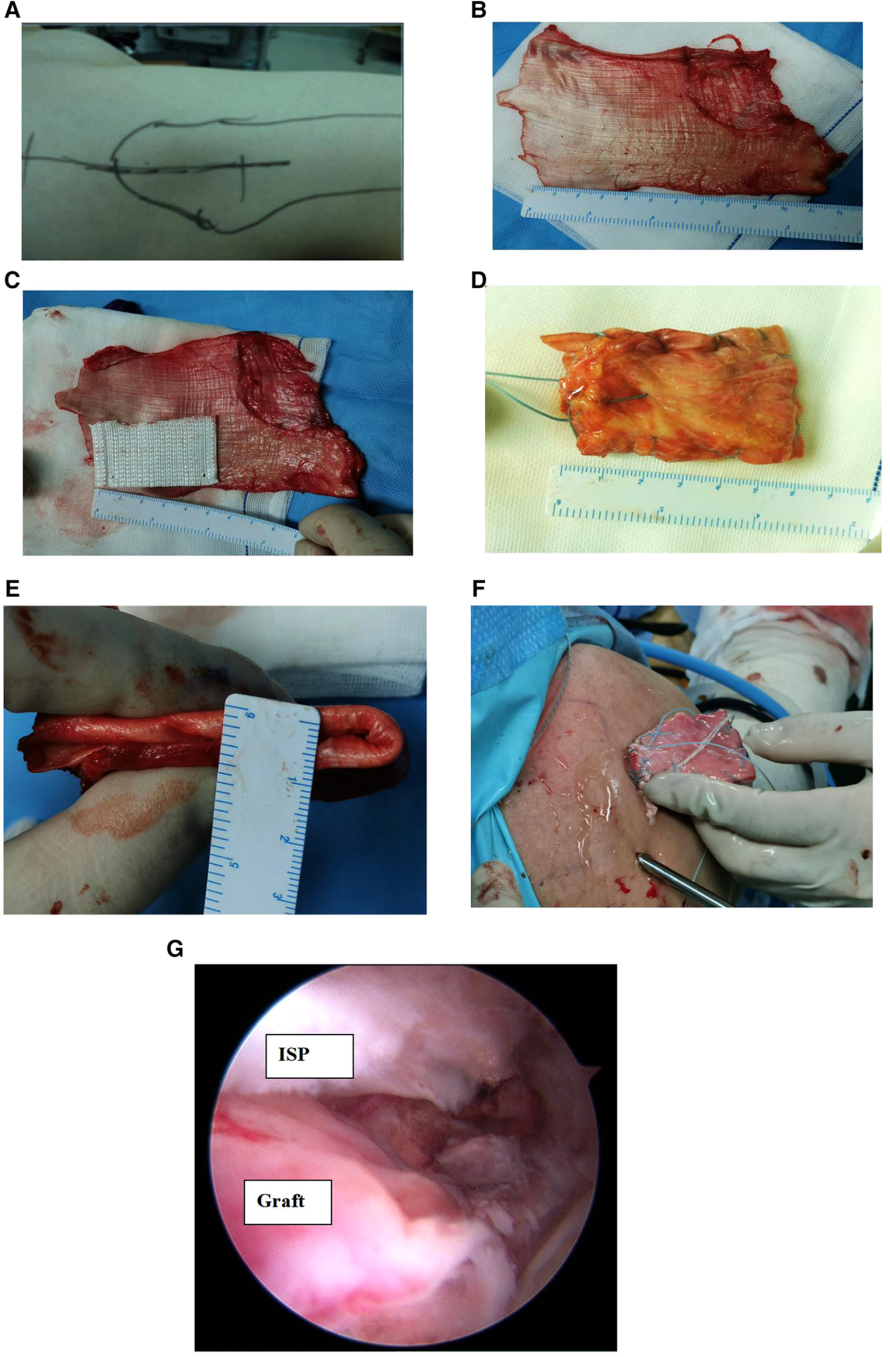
Intraoperative radiograph of arthroscopic superior capsule reconstruction. (A) Vertical skin incision; (B) harvested section of the fascia lata, two times the size of the superior capsular defect; (C) folding the fascia lata in half, combined with a pet material; (D, E) weaving the four sides with a non-absorbable suture to make a fascia lata patch, on which a graft 5.5–6.0 cm in length and 6–8 mm in thickness was fashioned by folding the fascia lata twice thick; (F) insertion of the fascia lata into the subacromial space through the anterolateral portal; (G) arthroscopic superior capsule reconstruction. ISP, infraspinatus tendon.
